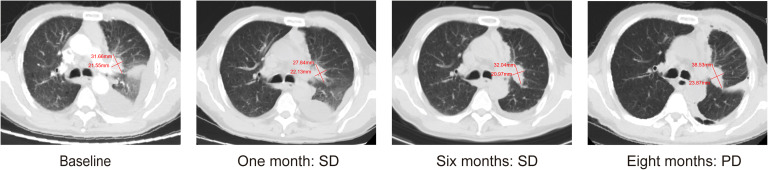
Representative computed tomography images of lung lesions.
